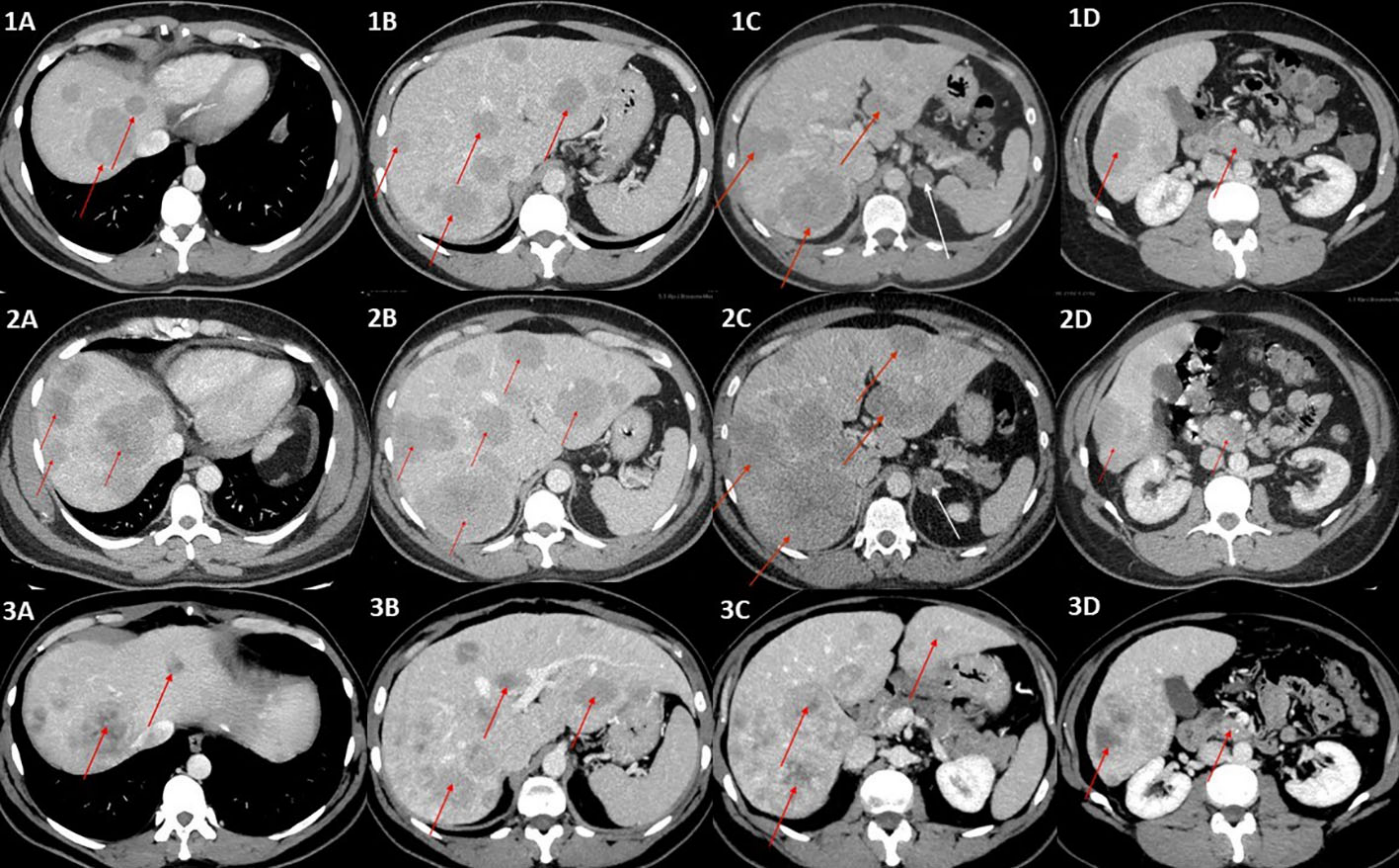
(1A–1D) Contrast-enhanced CT scans of the abdomen (portal–venous phase) showing multiple metastases in the liver, ranging in size from a few millimeters to 40 mm (red arrows), incidentaloma of the left adrenal gland of benign phenotype 20 mm in diameter (white arrow), and primary tumor (red arrow) (1D). (2A–2D) Contrast-enhanced CT (portal–venous phase) scans revealed progression of the metastatic liver disease (red arrows) and a significant change in the left adrenal tumor density (47 HU in native phase) and size (24 × 18mm), arousing suspicion of adrenal metastasis (white arrow), which was not confirmed in final pathology reports. The primary tumor is indicated by a red arrow in (2D). (3A–3D) Post left-sided adrenalectomy contrast-enhanced CT scan showing stable metastatic liver disease, without further progression in terms of intensity, number, and size of metastases, and even with some of the hepatic metastases shrinking (red arrows). The primary tumor is indicated by a red arrow in (3D).
